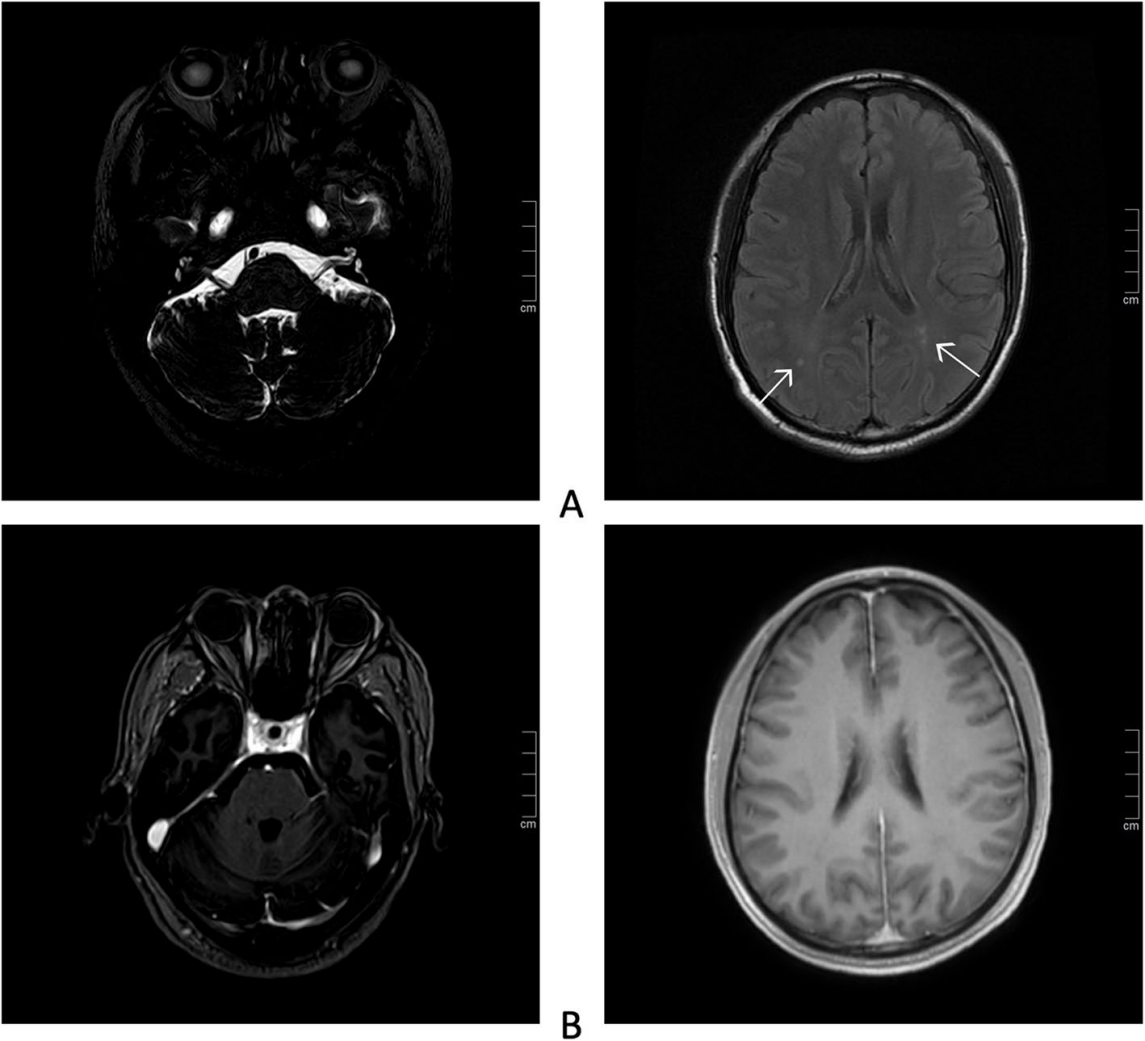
Brain MRI showed non-specific white matter changes but cranial nerve MRI was normal, with no observed in enhancement signal in contrast-enhanced scans. A Plain MRI of brain and cranial nerve. White arrows indicate non-specific white matter changes; B Contrast-enhanced MRI scan of brain and cranial nerve
